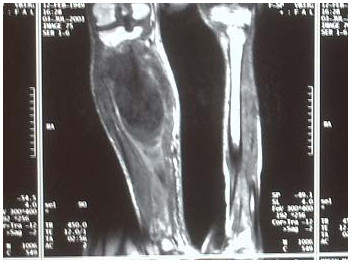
Magnetic resonance image scan demonstrating a saccular aneurysm of the right below-knee popliteal artery.
